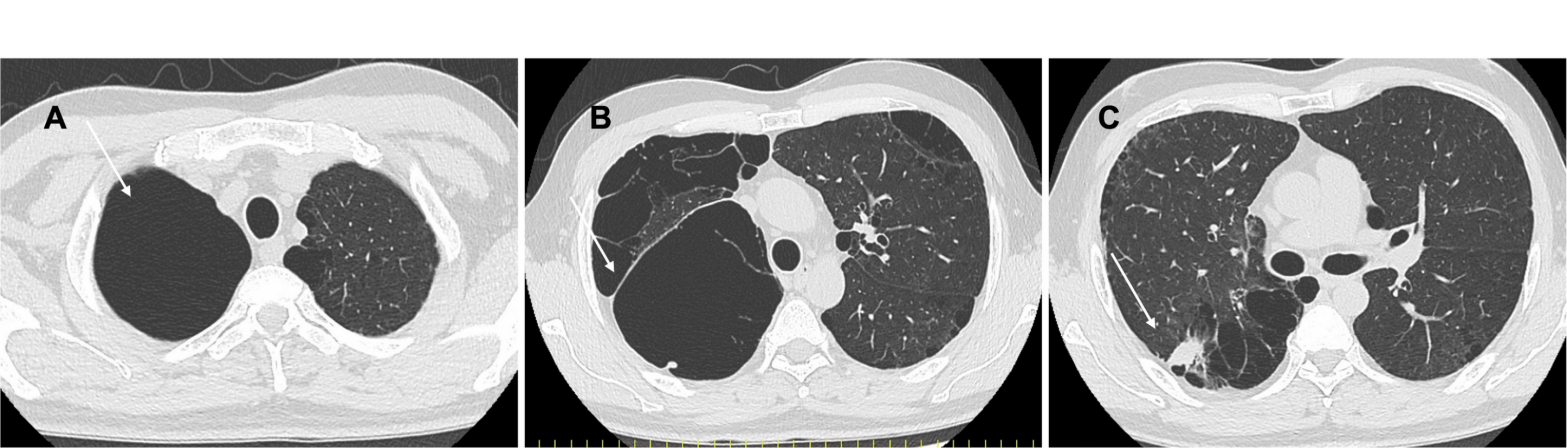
Chest computed tomography (CT) findings. A, B: Pulmonary cysts are present; C: A 15 x 14 mm irregular nodule was observed in the S2 upper lobe of the right lung.
